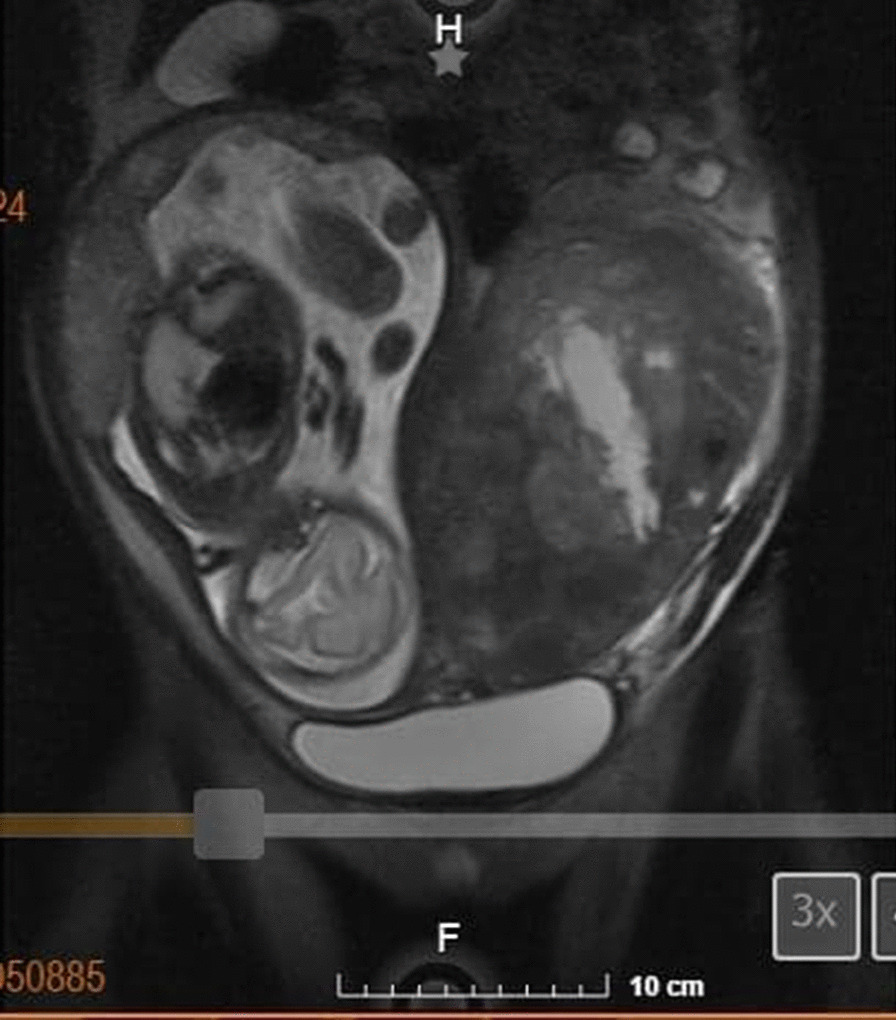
Abdominal-pelvic magnetic resonance imaging, T1 sequence. Pregnancy findings. Image showing one fetus in cephalic presentation, longitudinal lie, fetal dorsum to the left, with normal morphological findings, fundal-lateral placenta of regular margins, and homogeneous parenchyma. Left adnexal mass described in Fig. 2
